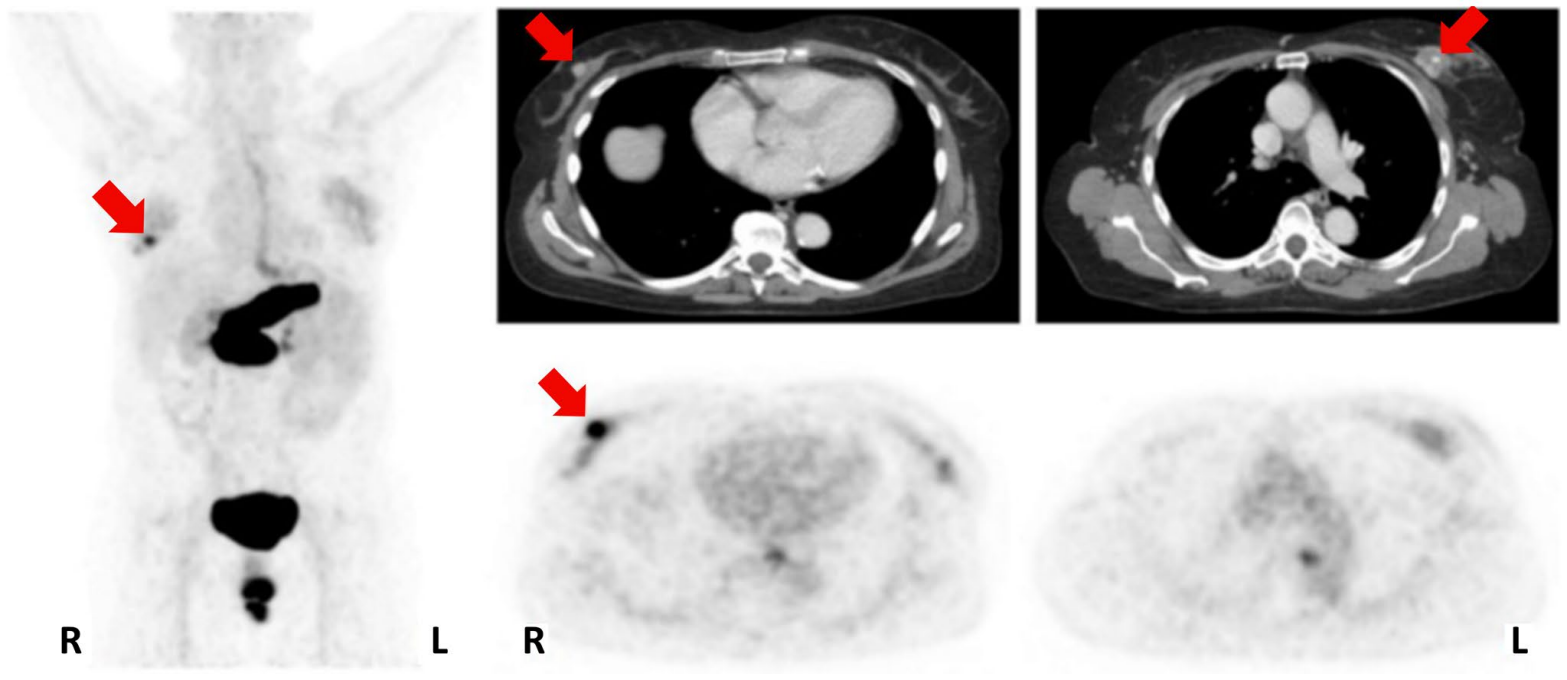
Patient with an ER/PR-positive tumor in the right breast (PET-positive; SUVmax 8.32) and an ER/PR-negative tumor on the left side (PET-negative; SUVmax 2.68). MIP left; CT upper row; [^68^Ga]Ga-RM2-PET lower row; primary tumors indicated by red arrows. Reprinted from Gastrin-releasing Peptide Receptor Imaging in Breast Cancer Using the Receptor Antagonist 68Ga-RM2 and PET by Stoykow et al. (89), under Creative Commons Attribution (CC BY-NC) license.
